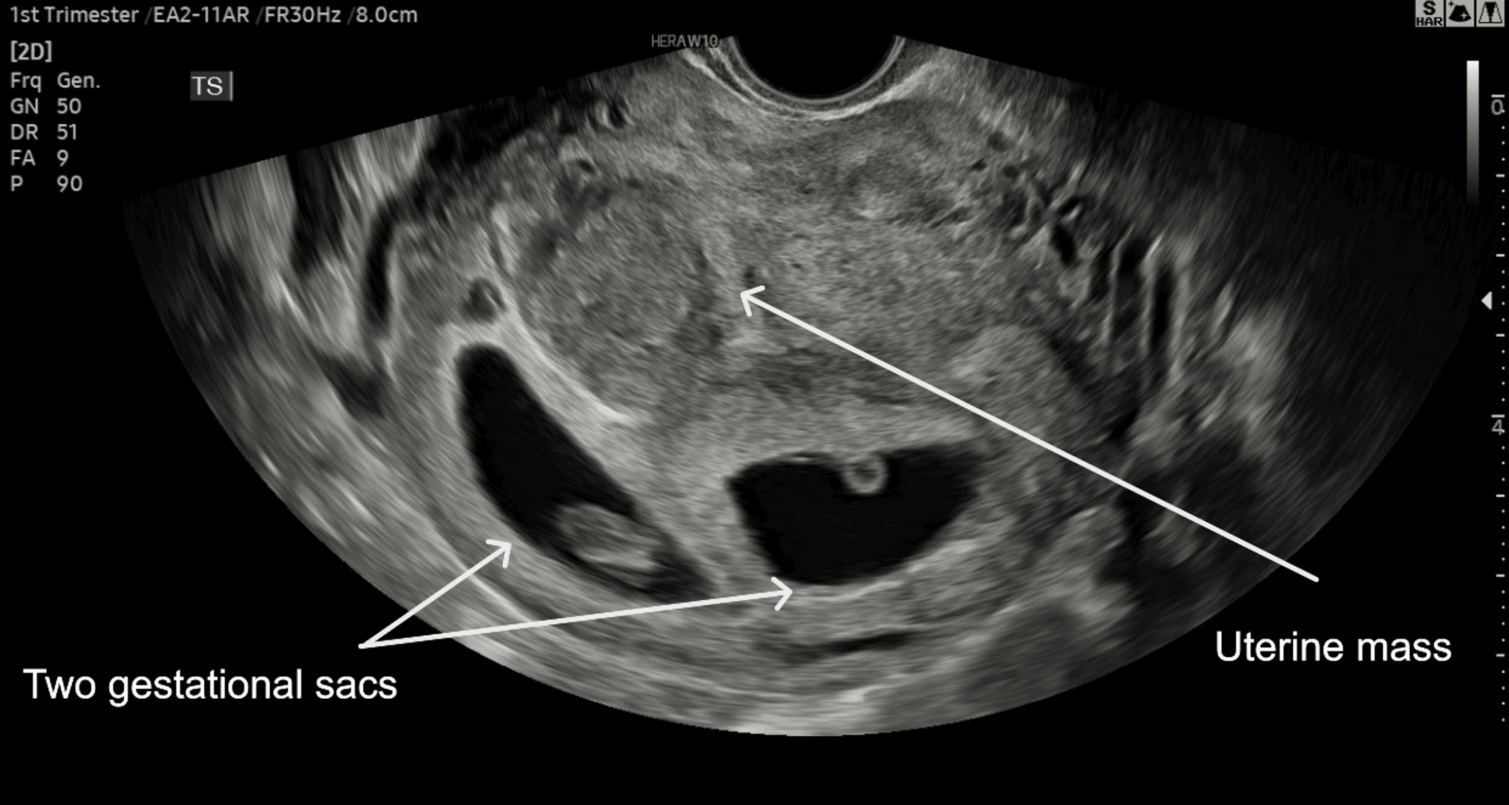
Ultrasound at seven weeks of gestation showing twins and the same uterine lesion
